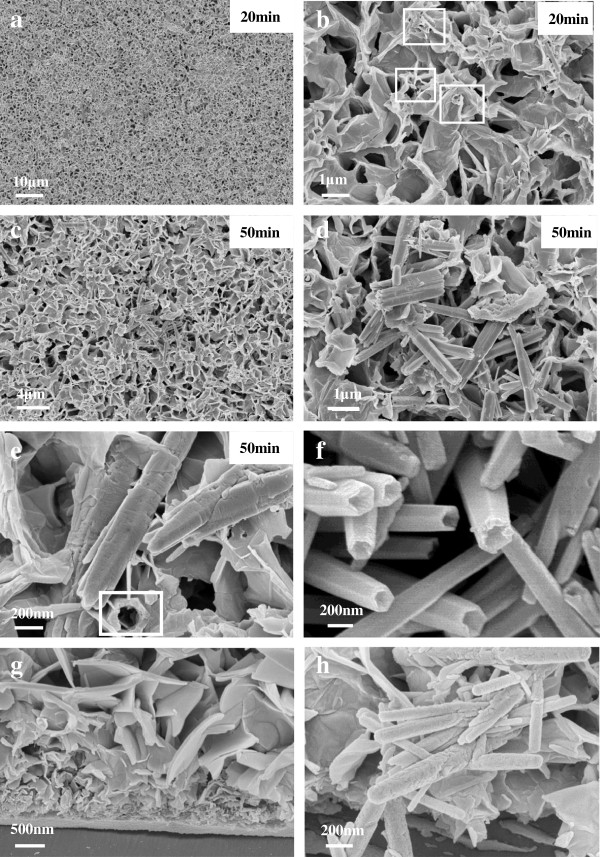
ZnO sheet networks formed on an Al foil upon ultrasonication. Low (a), high (b) magnification SEM images of ZnO on Al foils after 20 min ultrasonication vibration, (c, d, e) SEM images of ZnO on Al foils after 50-min ultrasonication vibration, (f) SEM images of ZnO on Al foils after 50 min ultrasonication vibration, (g, h) cross-sectional SEM images of the sample before and after ultrasonic treatment.
